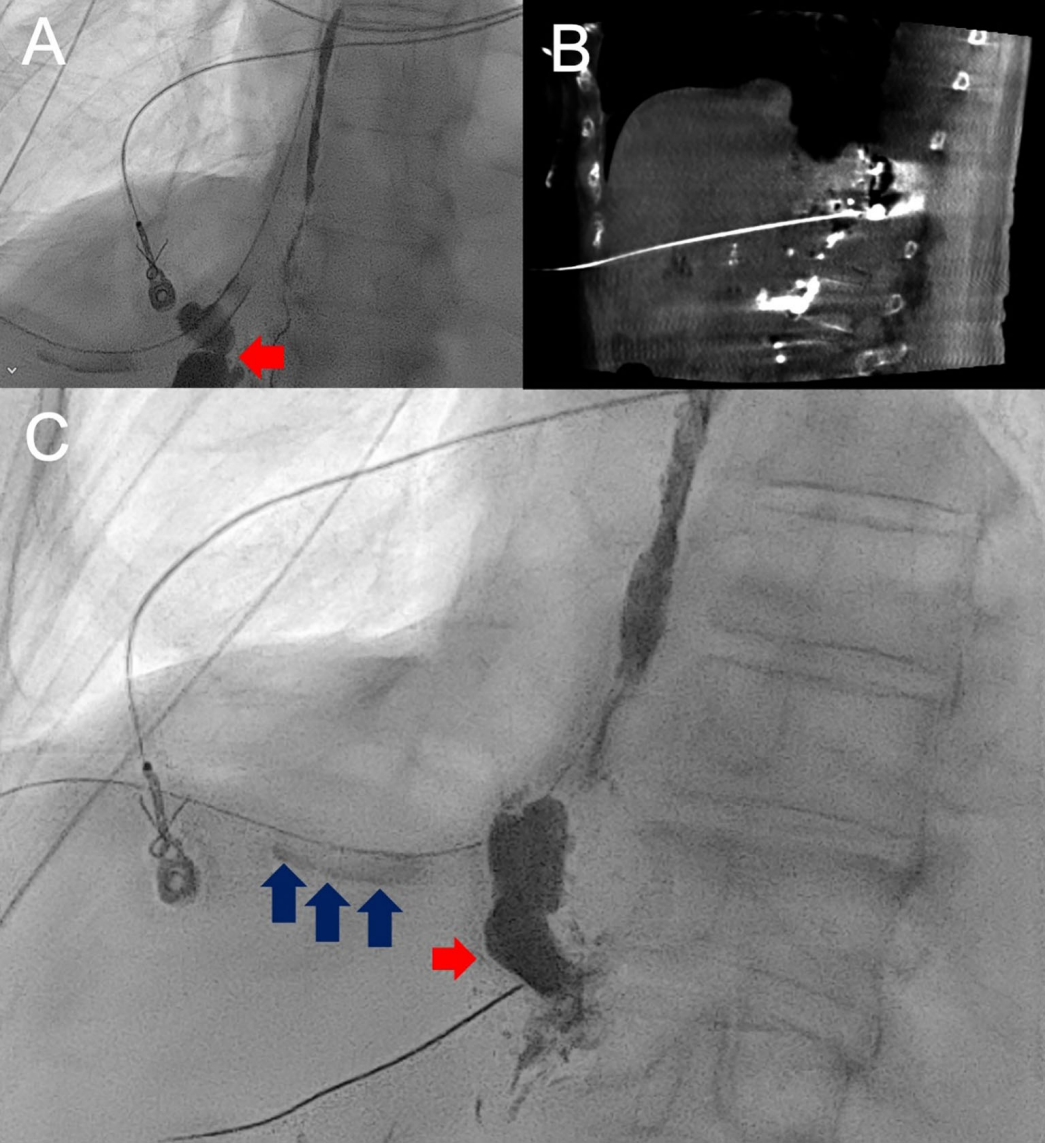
AP fluoroscopic image and lymphangiogram of Patient 3 with lipiodol opacified thoracic duct and extravasation overlying the right lower hemithorax (red arrow) (A). Following unsuccessful catheter and wire manipulation to the known site of leak, cone-beam CT-guided,anterior-approach transhepatic direct stick (B) of the leak with subsequent lipiodol injection confirmed leak with visualization of lipiodol passage through the right-sided chest tube (blue arrows) followed by glue embolization (C).
